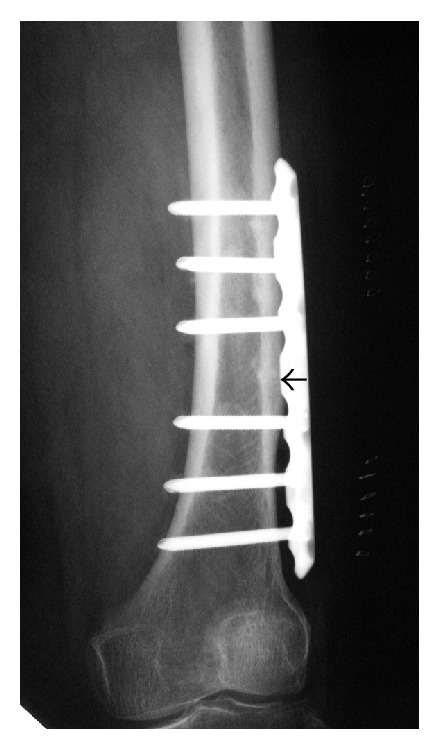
Anteroposterior radiograph of the left femur treated surgically using a locking plate. Black arrow shows original horizontal line.
